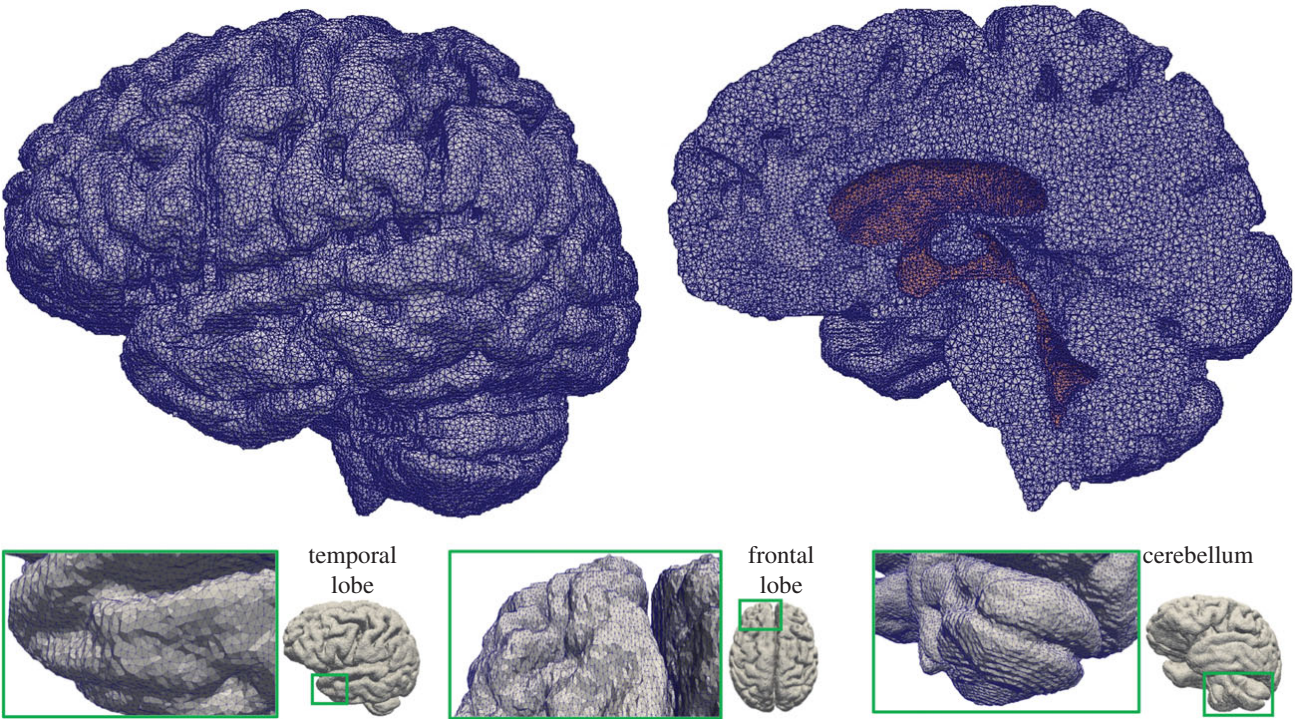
Tetrahedral mesh of the control subject generated from T1w-MR images. The figure on the top right-hand side shows a cross section of the volumetric mesh, where the dark area represents the ventricular wall. The number of tetrahedral elements in this case is 2 772 075. (Online version in colour.)
